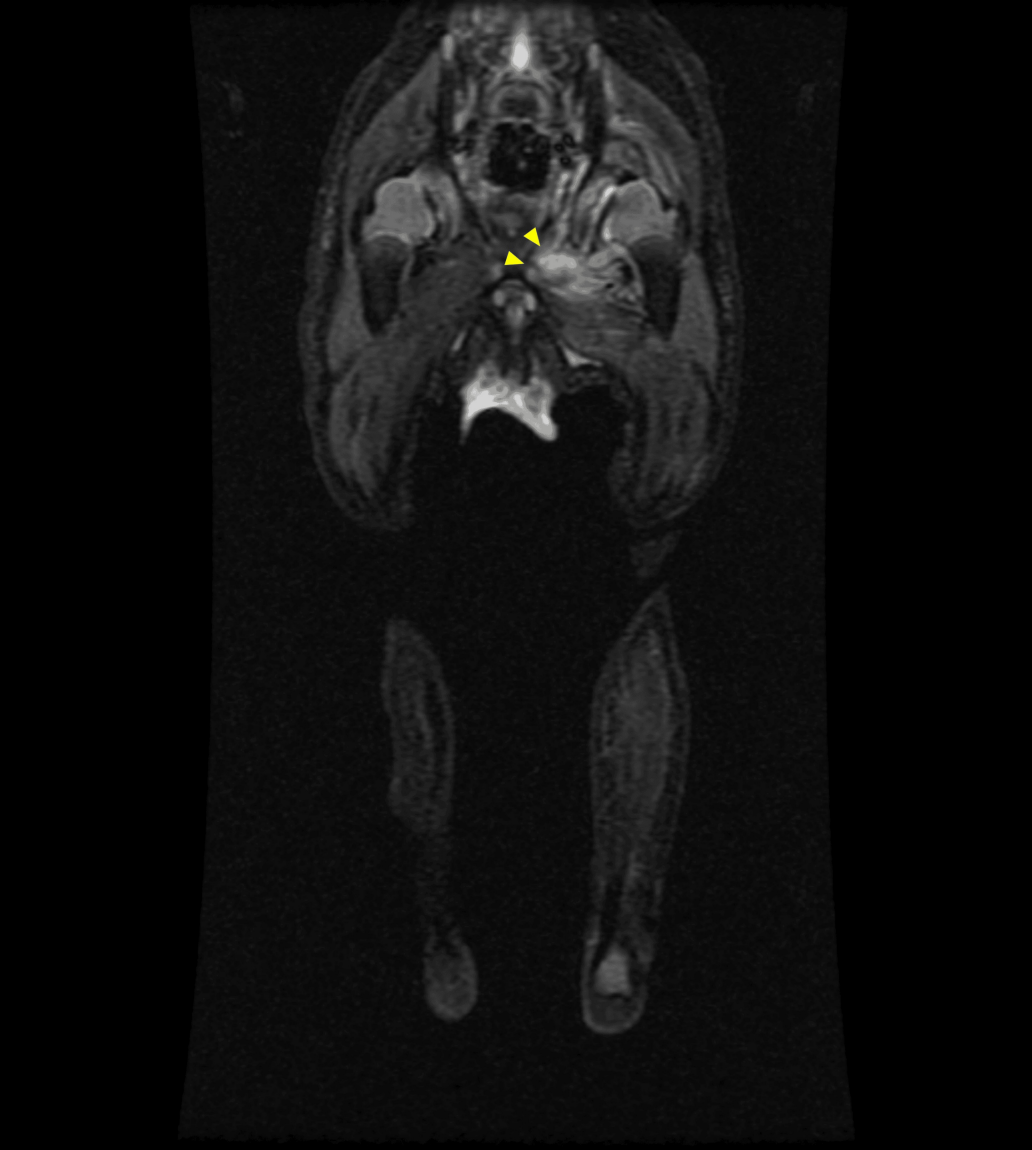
Coronal T2-weighted MRI of the left hip. Marked high signal intensity is observed involving the left pectineus and adductor musculature, suggestive of myositis (yellow arrowheads).
